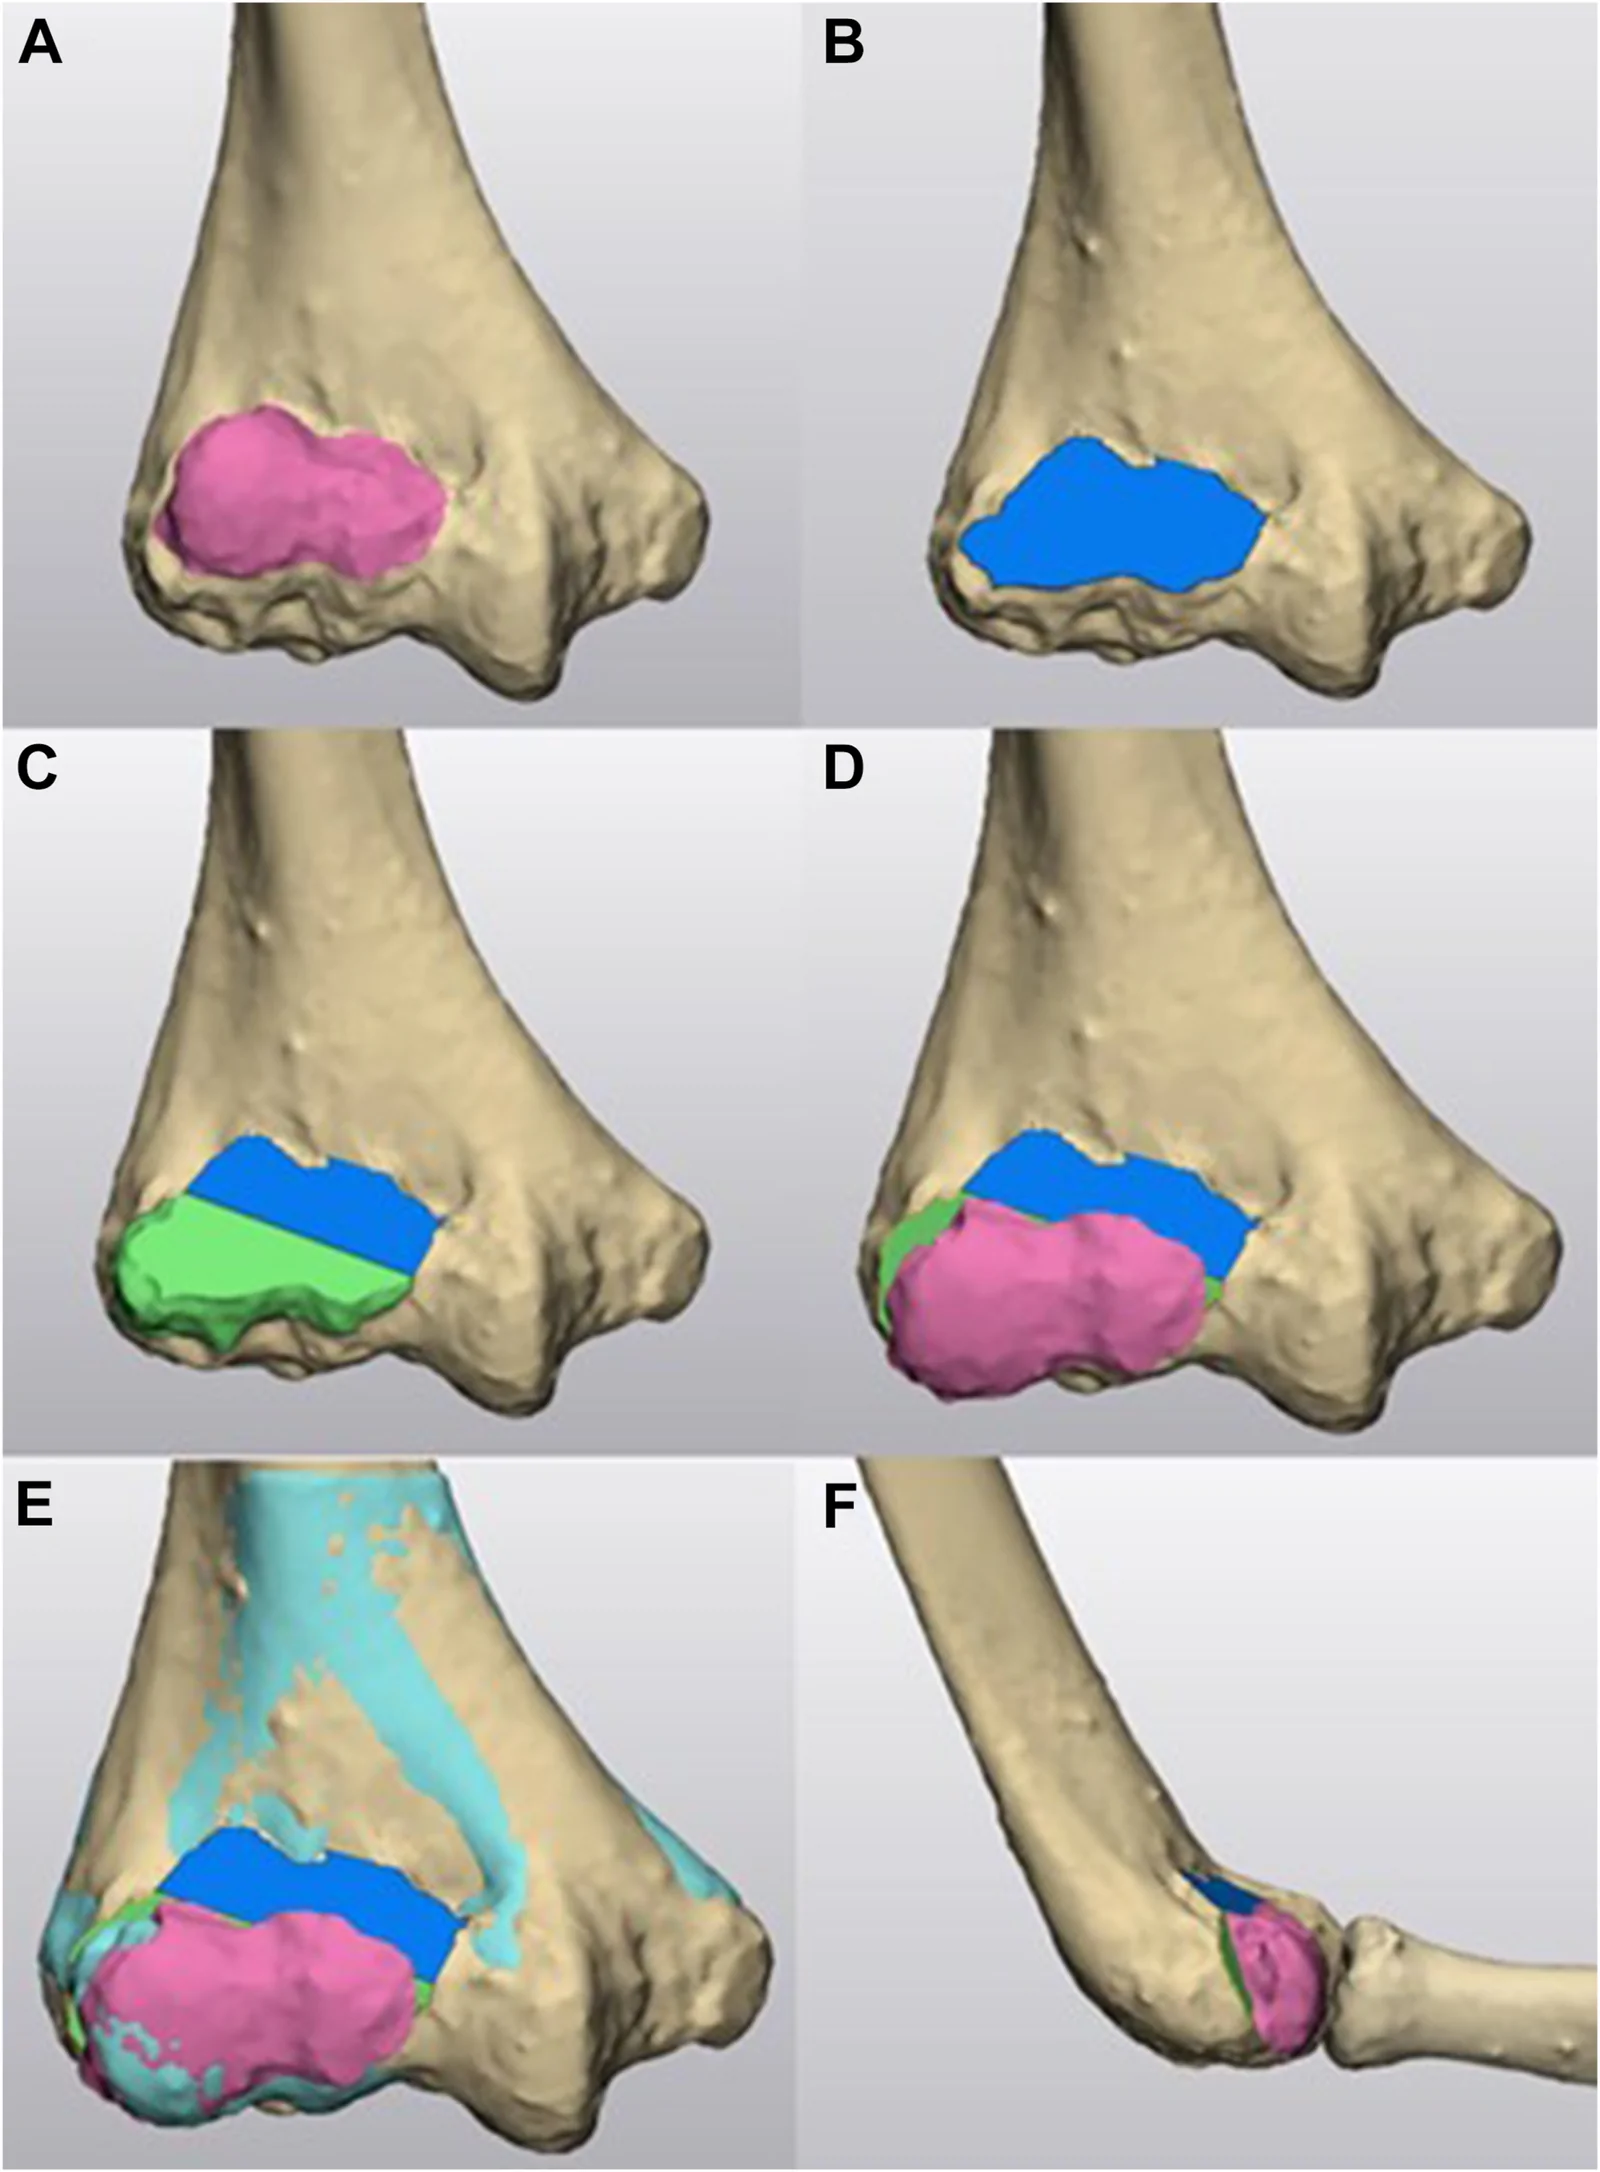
Case 2 - Pre-operative 3D reconstruction of the elbow joint based on CT scans. (A) The position of the malunited capitellum. (B) Highlighted in blue, the elbow joint after osteotomy of the capitellum. (C) In green, the additional bone to be resected before the reduction. (D) Reduction of the capitellum according to the 3D reconstruction. (E) Comparison with the contralateral distal humerus (light blue) after reduction. (F) Post-operative alignment relative to the proximal radius. *3D*, three-dimensional.
